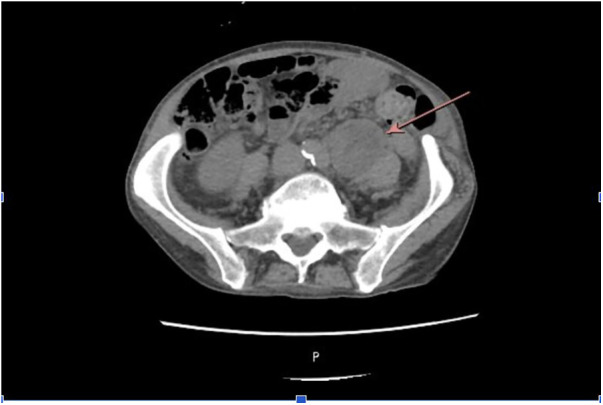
Initial diagnostic CT (November 2023). Legend: Axial contrast-enhanced CT of the abdomen and pelvis obtained at initial presentation (November 2023), demonstrating a well-circumscribed soft tissue mass (arrow) that prompted further diagnostic workup.
